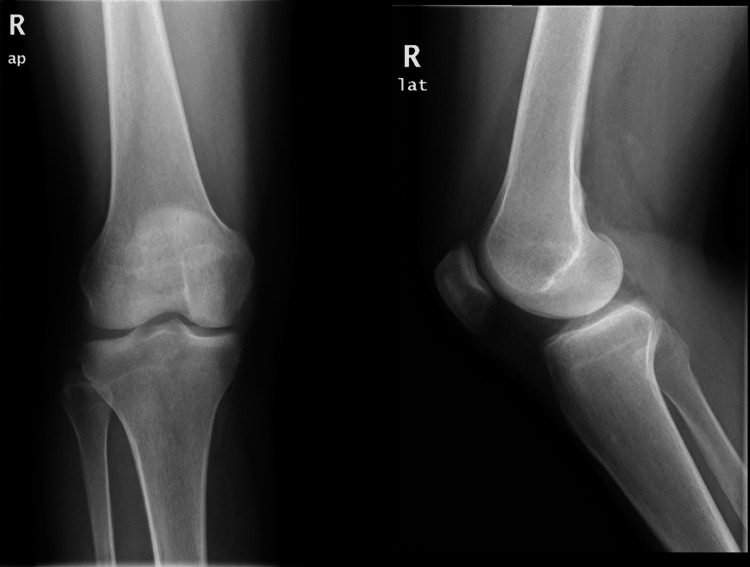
Anteroposterior and lateral X-ray views of the knee. No abnormalities were detected in the bone and soft tissues.
